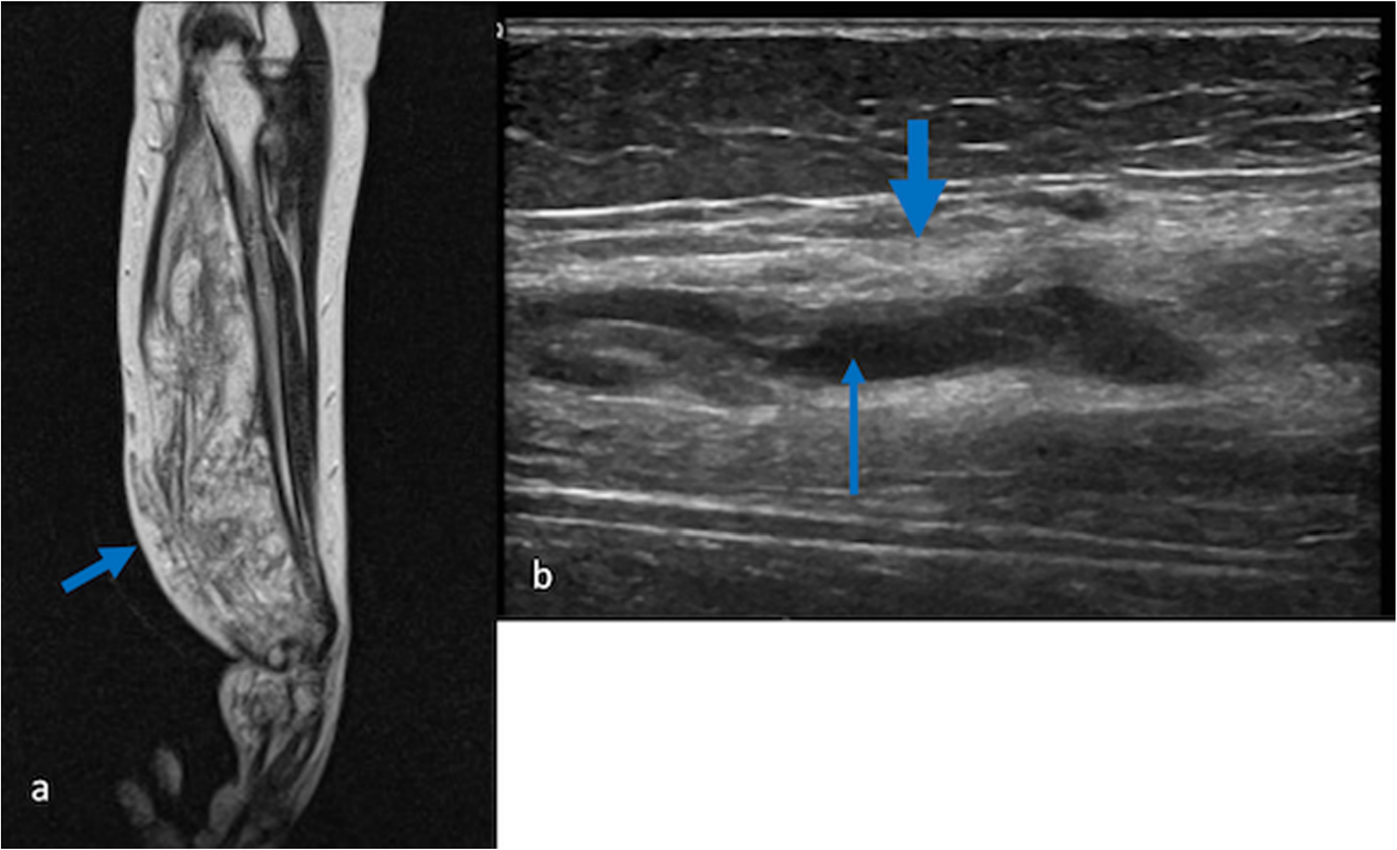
a sagittal T1 weighted MR image of the forearm in a 12 year old female. The FAVA lesion (short arrow) replaces almost all of the flexor compartment and is causing contracture at the wrist; b US image of a FAVA lesion of the calf, showing marked echogenicity of the affected muscle (thick arrow) and central dysplastic veins (thin arrow)
